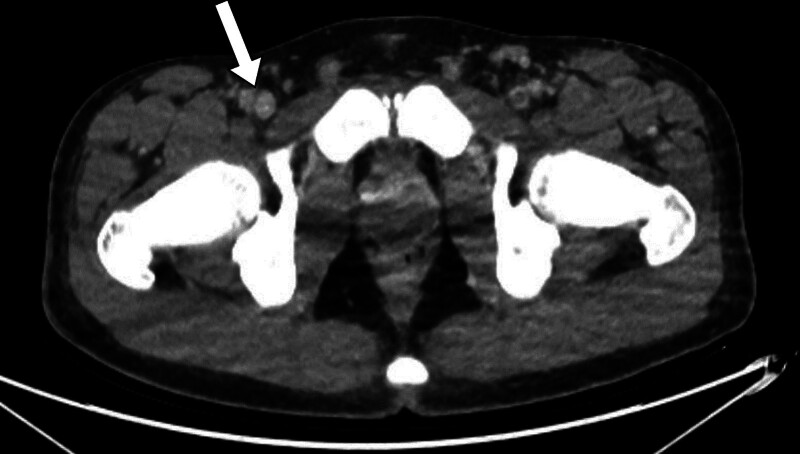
CT image of post-anticoagulation. Right common femoral vein thrombus resolution. CT = computed tomography.
